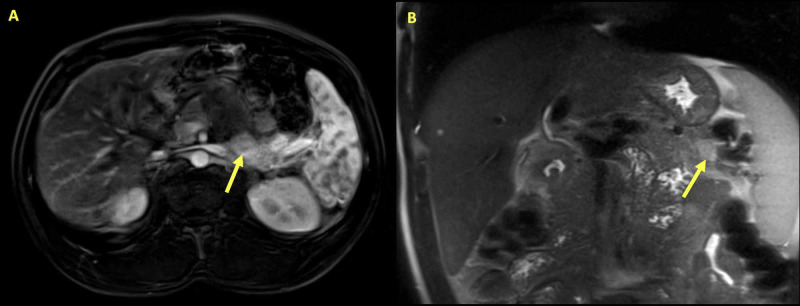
Abdominal Magnetic Resonance Imaging (A) T1-weighted axial section and (B) T1-weighted coronal section of abdominal magnetic resonance imaging with arrows highlighting a 2.8 cm x 1.3 cm area of variable enhancement and diffusion restriction within the pancreas, consistent with insulinoma.
